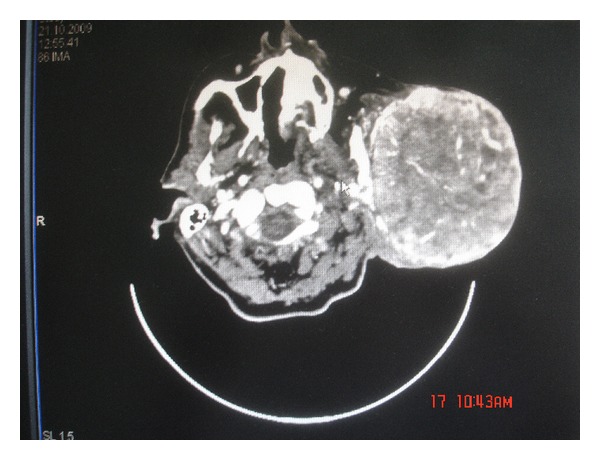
Postcontrast CT scan of the tumor.
